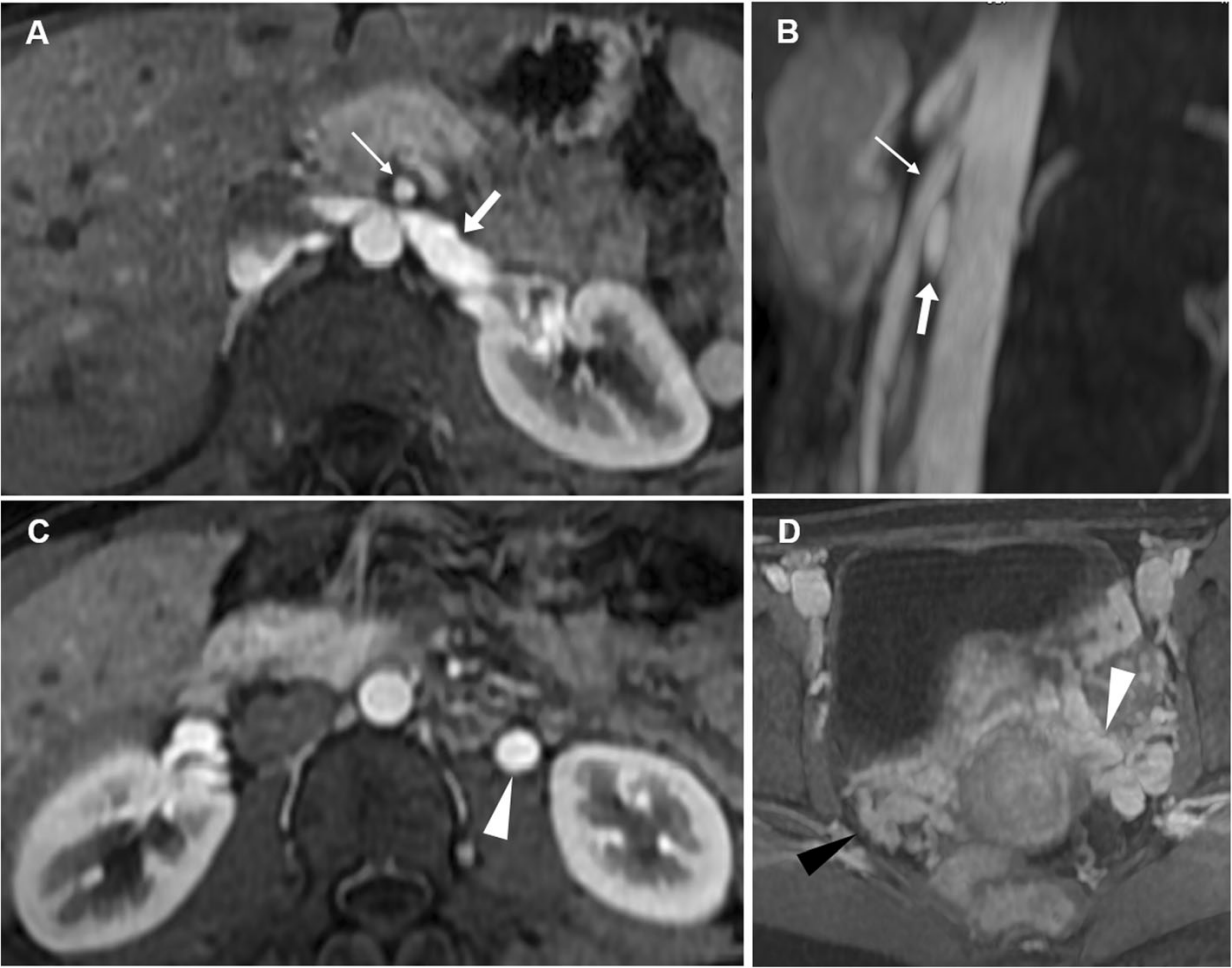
Pelvic varices from nutcracker syndrome in a 24-year-old woman with severe pelvic pain. Axial gadolinium-enhanced fat-suppressed T1-weighted images (a, c) demonstrate compression of left renal vein (‘beak sign’, arrow) between the abdominal aorta and superior mesenteric artery (thin arrow) and enlarged left gonadal vein (arrowhead). Additional sagittal maximum-intensity projection (MIP) reconstruction (b) demonstrates the left renal vein (arrow) within a narrow angle between the abdominal aorta and the superior mesenteric artery (thin arrow), consistent with ‘nutcracker syndrome’. Axial angiographic MIP image (d) demonstrates dilated, engorged left parametrial and uterine veins (arrowhead). Note the dilatation of contralateral parametrial plexus (black arrowhead) due to the anastomosis via the arcuate uterine veins
